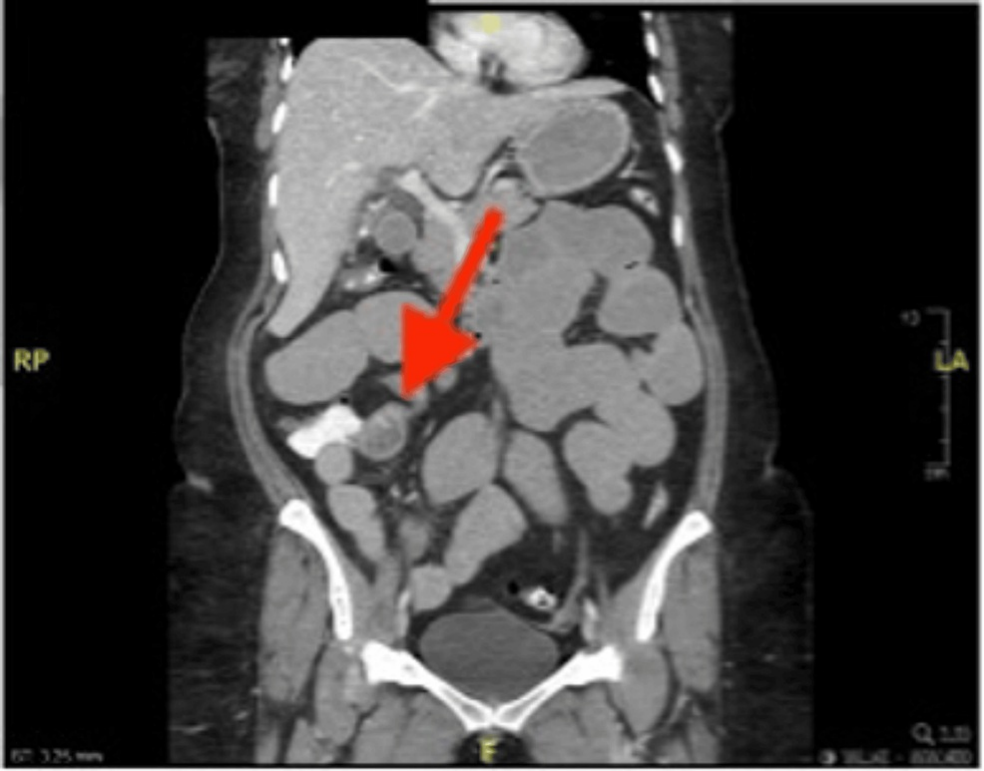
Coronal CT scan with contrast demonstrates the gallstone causing the ileus
